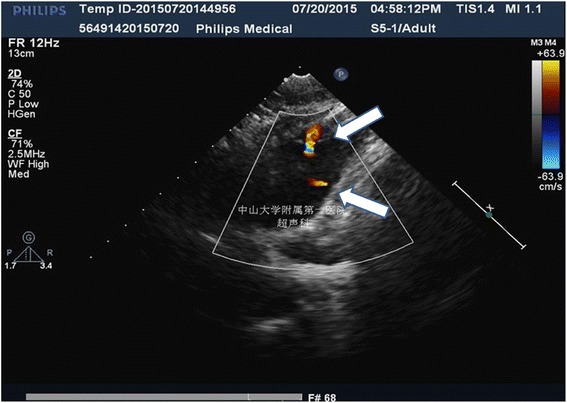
Preoperative echocardiography showed two abnormal transverse shunts in pulmonary trunk. One was 1.6 mm wide (*upper arrow*) and the other was 0.6 mm wide (*lower arrow*) which were both considered as coronary to pulmonary artery shunts
